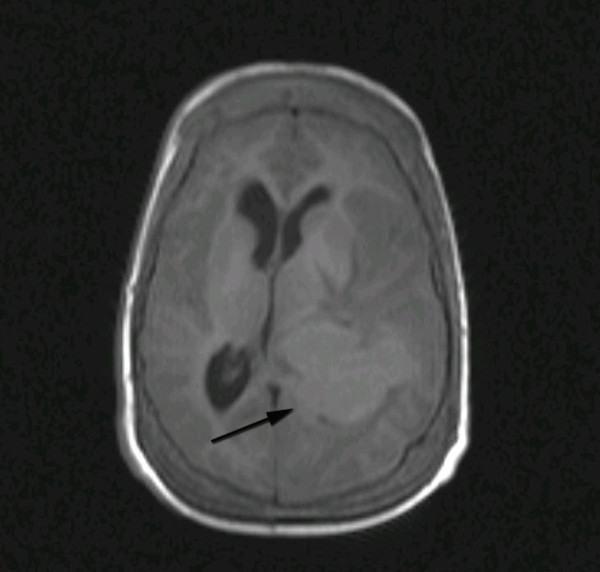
Axial T1-weighted MRI image demonstrating a well-demarcated isointense lesion in the left trigone with significant surrounding edema and mass effect.
